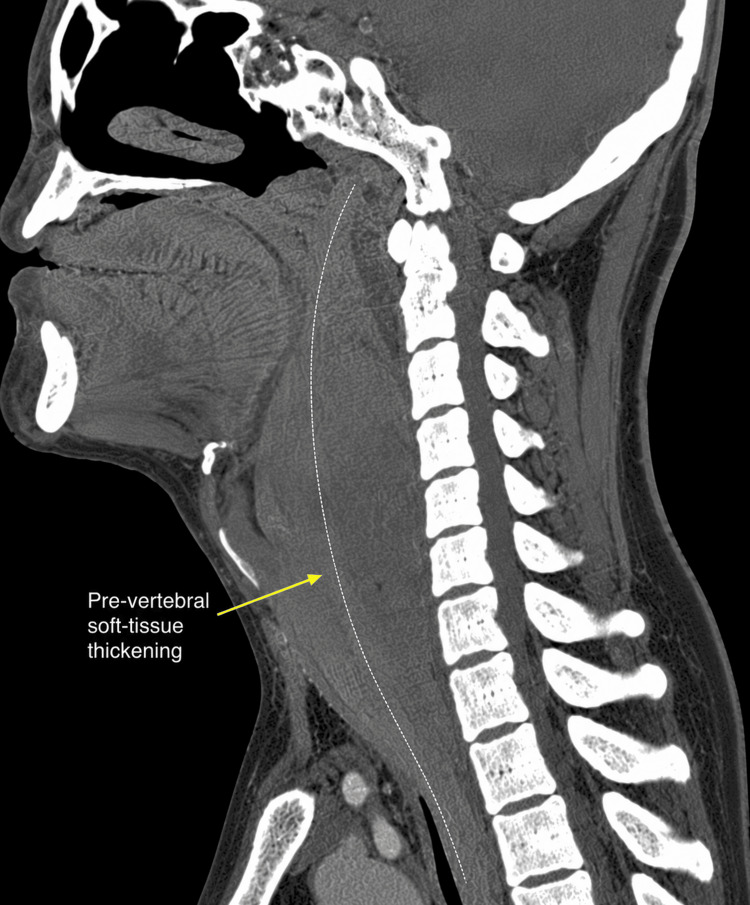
Pre-vertebral soft tissue thickening on sagittal view of the CT scan of neck
